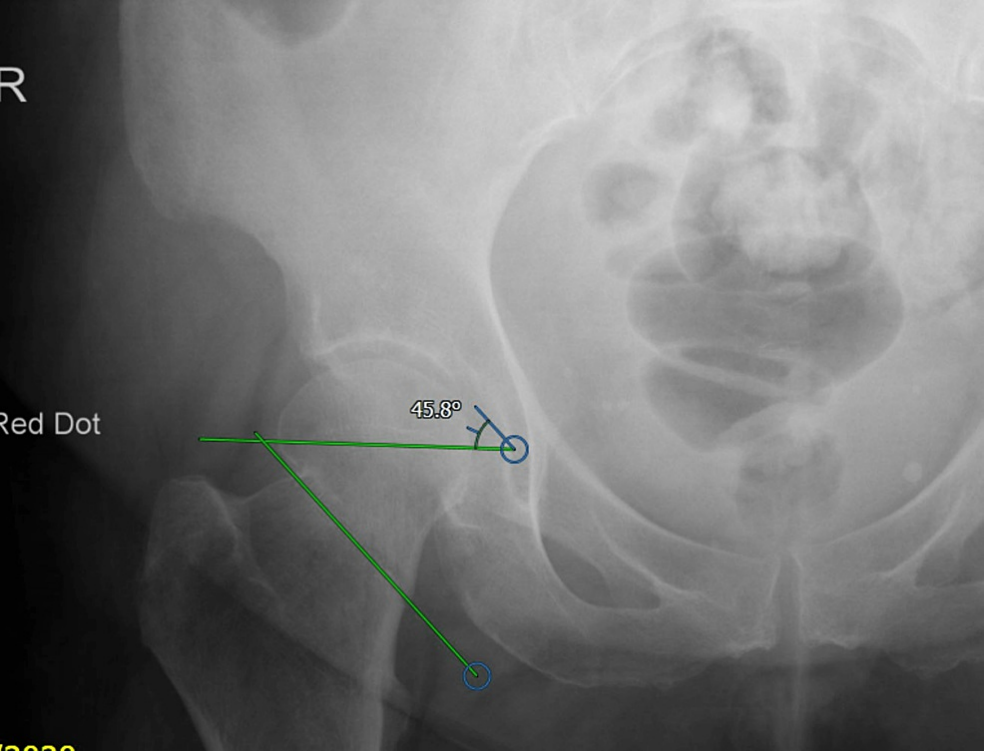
AP X-ray of the hip with NOF fracture (Pauwels 2) AP, anteroposterior; NOF, neck of femur
